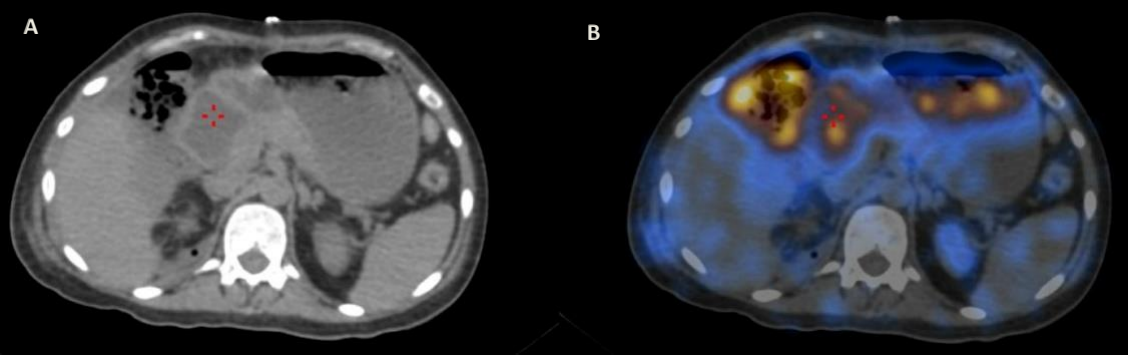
(A) Non contrast CT and (B) Fused SPECT CT images showing the collection of tracer in the perirenal space, paracolic gutter, and perihepatic spaces
